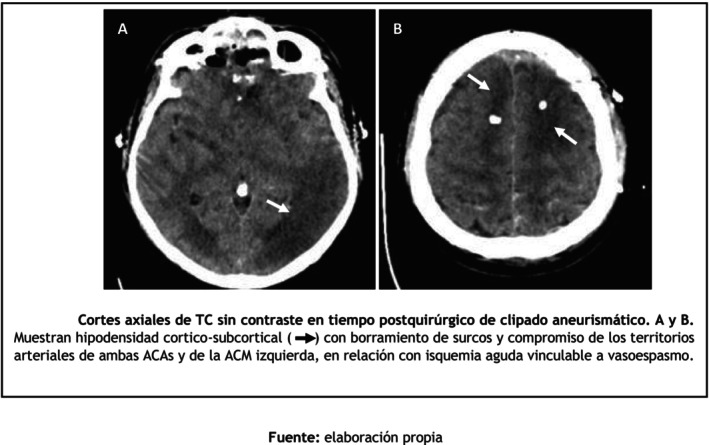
Postoperative non‐contrast axial brain CT images after aneurysm clipping. (A, B) Cortical‐subcortical hypodensity (
